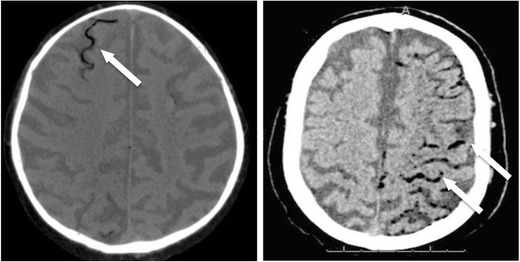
Post-procedural CT scan after chest biopsy showing two different patients with air embolism in cerebral vessels (arrows)
